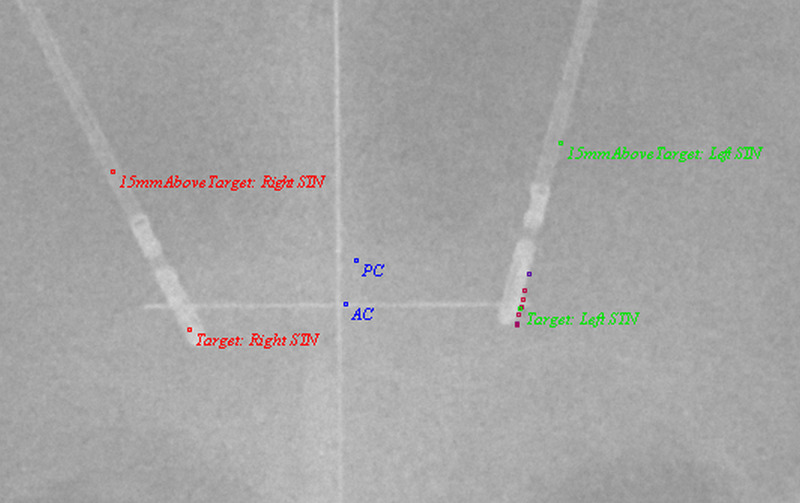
AP X-ray image showing kinesthetics acquired in 3D space and mapped to the 2D image on the left side. Note the slight disparity between MER tract, with which the kinesthetic points were acquired, and the final DBS electrode position. AP = Antero-Posterior; AC = Anterior Commissure; PC = Posterior Commissure; STN = Subthalamic Nucleus; DBS = Deep Brain Stimulation; MER = Microelectrode recording; 3D = Three Dimensional; 2D = Two Dimensional.
